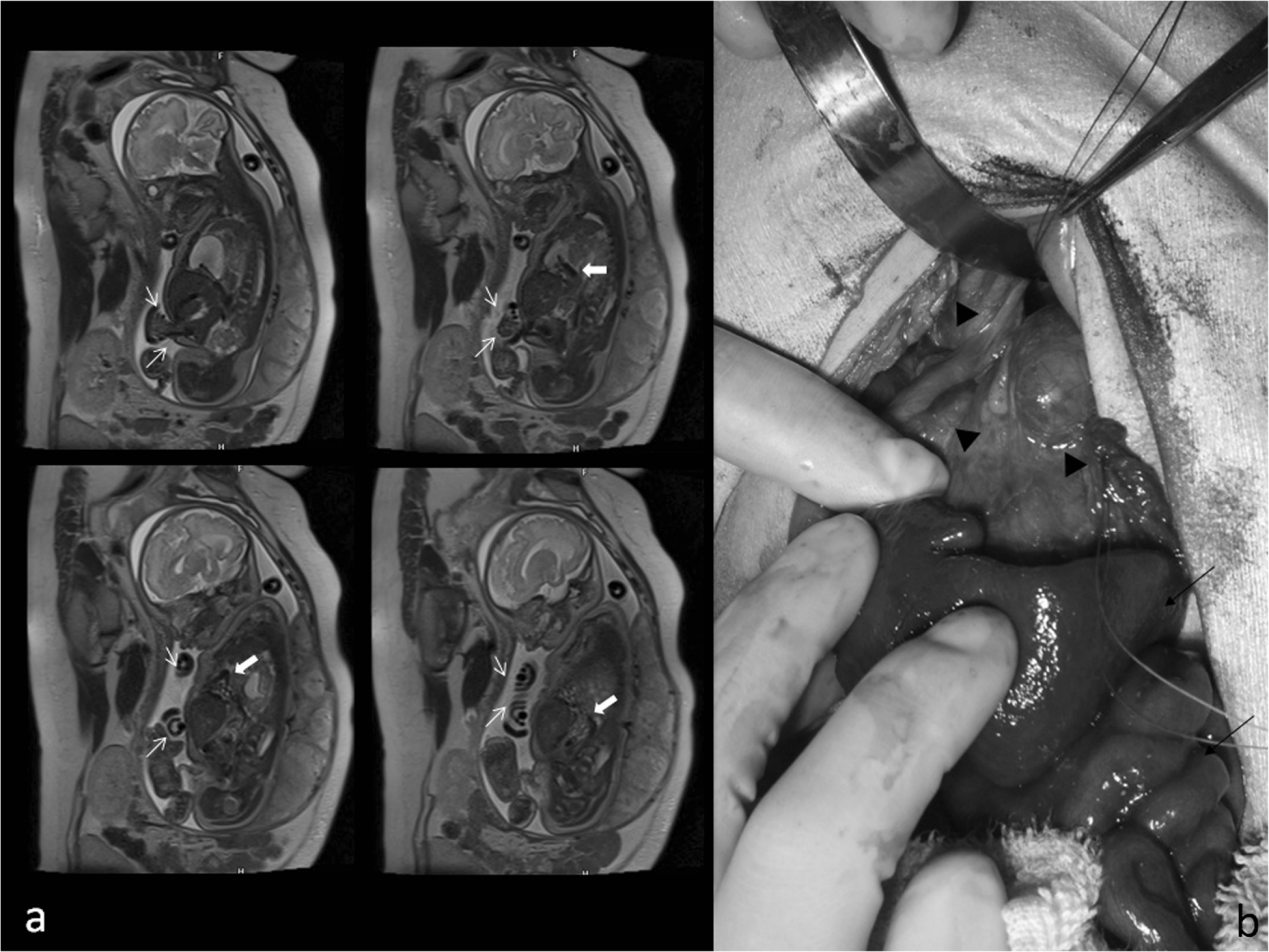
Fetal magnetic resonance imaging (a) and photograph at surgery (b) of Case 1. a Herniation of abdominal organs into the left pleural cavity (➩) and intestine into the umbilical cord (↓) were detected by magnetic resonance imaging at 34 weeks of gestation. b The spleen and small intestine were removed from the left pleural cavity and reduced into the abdomen (↓). Anterior, posterior, and left lateral posterior sides of diaphragm were formed but mediastinal side, especially around the esophageal hiatus of diaphragm, was defective (▼)
